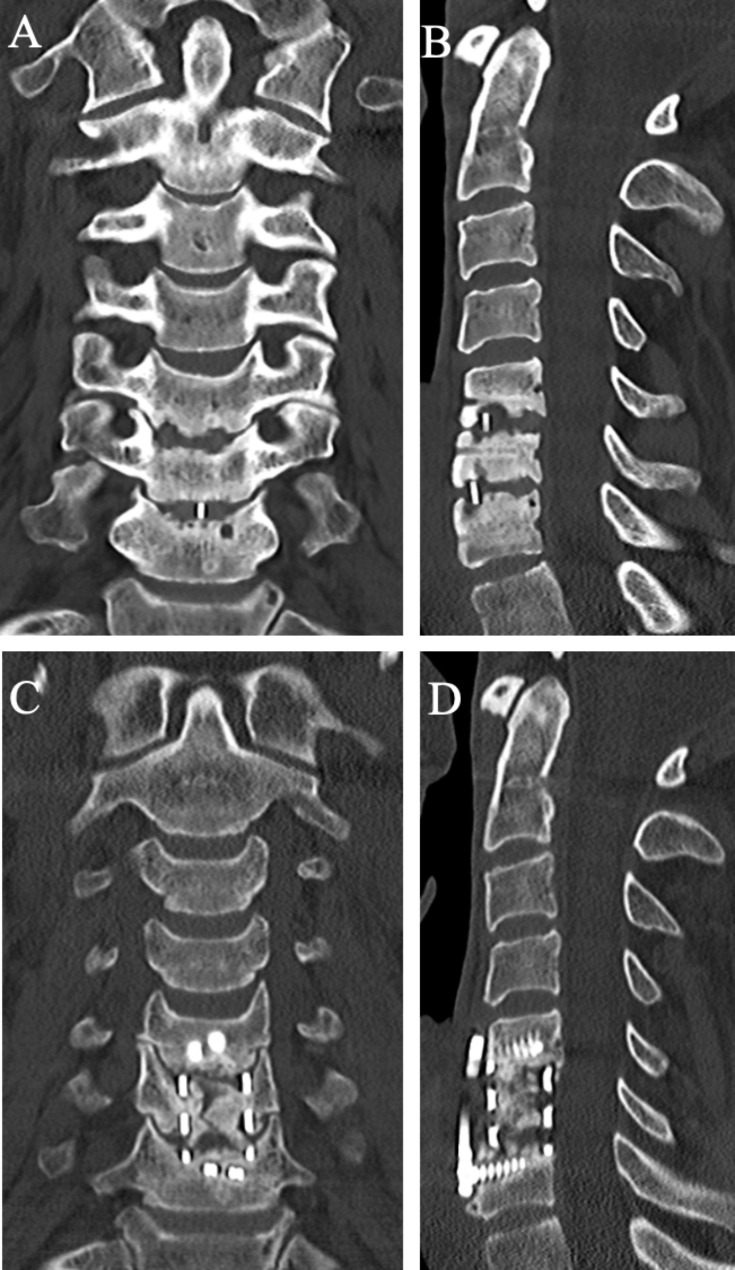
Postoperative coronal and sagittal CT two years after the index surgery shows pseudoarthrosis at C5/6 and C6/7 (A + B). Coronal and sagittal postoperative CT one year after revision surgery shows persistent pseudoarthrosis (D + E)
